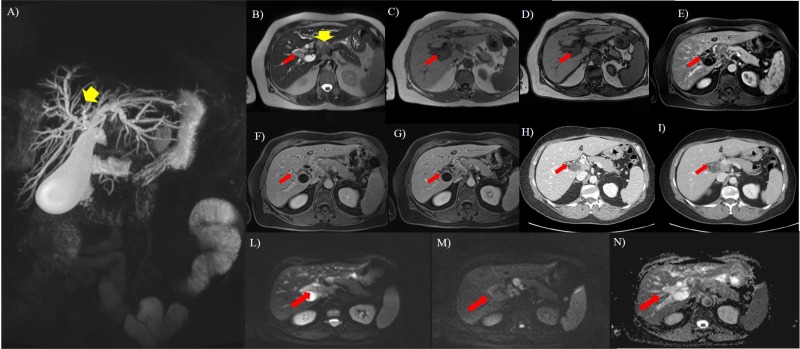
Seventy-three years old woman with breast cancer. A, MIP image. Common hepatic duct is not evident (yellow arrow) and, this is due to a hilar lymph node (yellow arrow) detect in B (axial T2-W HASTE). In b it is also evident the presence (red arrow) of peribiliary tissue as a hyperintense mass extending to the bifurcations of both right and left hepatic ducts. In C (axial T1-W in phase) and D (axial T1-W out phase) the lesion appears as hypointense. After contrast medium (E, arterial, F, portal, G, equilibrium phase) the lesion is not clearly evident so as in arterial phase (H) and portal (I) phase on CT study. DWI sequences: in L *b*50 s/mm^2^, in M *b*800 s/mm^2^, in N ADC map. The tumor (red arrow) shows restricted diffusion.
